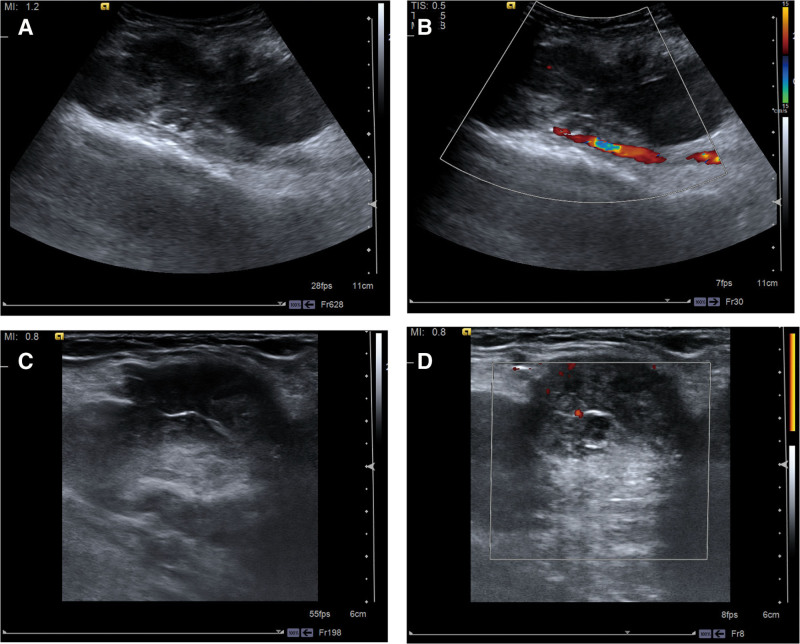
Ultrasound showed a heterogenous mass in the right axillary: (A) Gray-scale sonography of the right axillary evaluated by convex probe; (B) Color Doppler showing an arterial vessel nearby the mass; (C) Gray-scale sonography of the right axillary evaluated by linear probe; (D) Power Doppler shows the presence of vessels within the mass.
